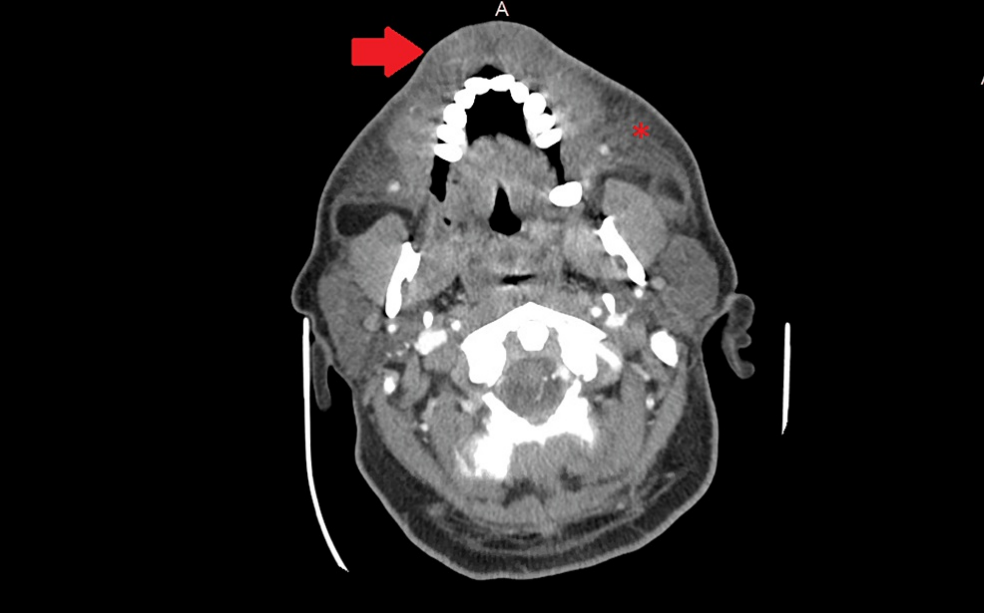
CT neck axial view highlighting subcutaneous edema. The red arrow shows massive enlargement of the upper lip while the asterisk highlights the diffuse involvement of the premaxillary soft tissues.
